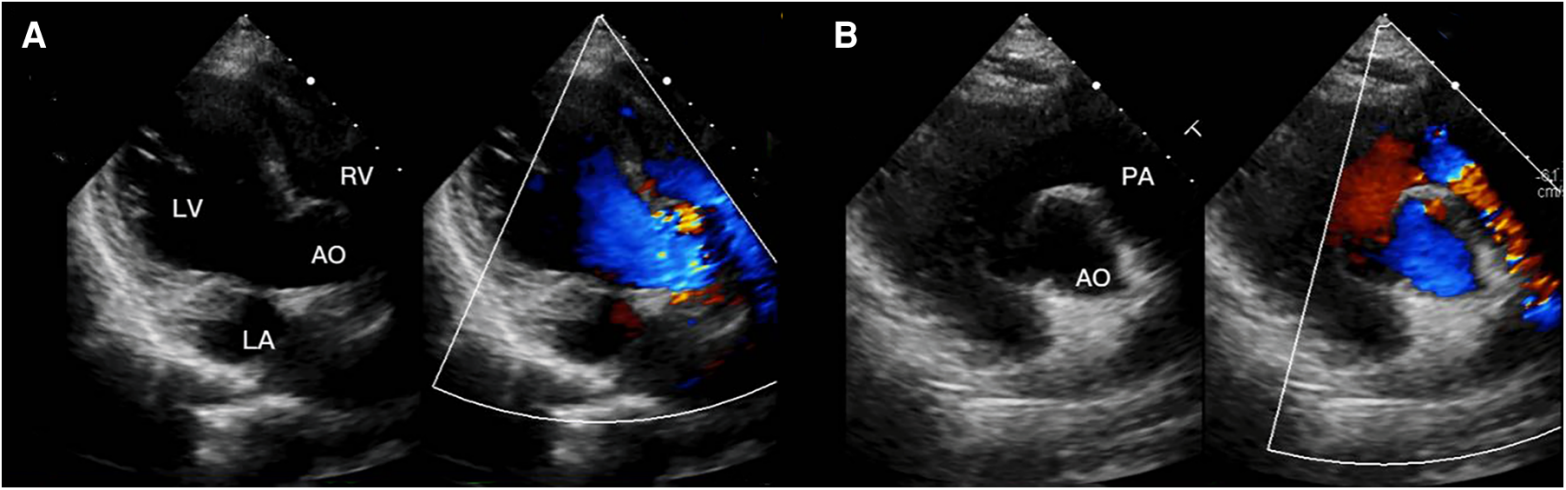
(A) transthoracic echocardiogram 3 months after surgery in four-chamber view of the right subcostal arch, (B) transthoracic echocardiogram 3 months after surgery in right parasternal short axis view.
